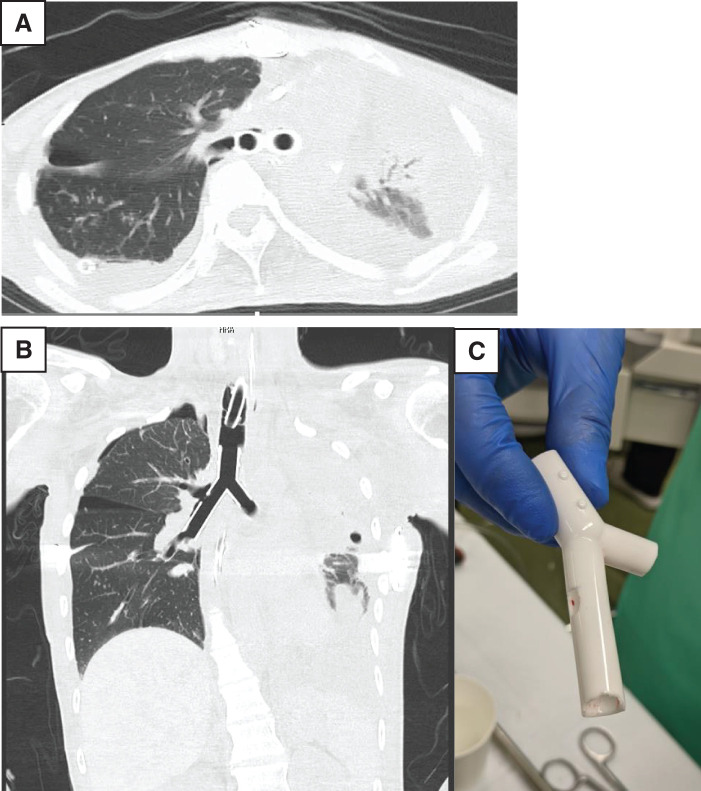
(A, B) Chest CT after stent placement: A TRACHEOBRONXANE DUMON Y-stent was implanted into the bronchial lumen without branch occlusion. (C) DUMON Y stent was trimmed to avoid blockage of the right upper lobe bronchus and mid-lobe bronchial inlet. TRACHEOBRONXANE DUMON Y-stent, Novatech SA, La Ciotat, France
